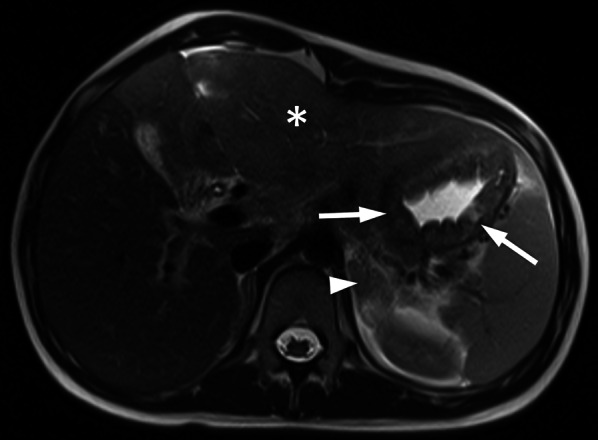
A 15-year-old girl with gastric adenocarcinoma (signet ring cell carcinoma). She had abdominal pain and anemia complaints for the last 4–5 months. Her Hb value was 6.9 gr/dL (normal range 11.7–15.5 gr/dL). Axial T2-weighted image shows diffuse increased gastric wall thickening (arrows) and diffuse hyperintense heterogeneous metastases at the left liver lobe (asterisk). Note the left adrenal mass consistent with metastasis (arrow head)
